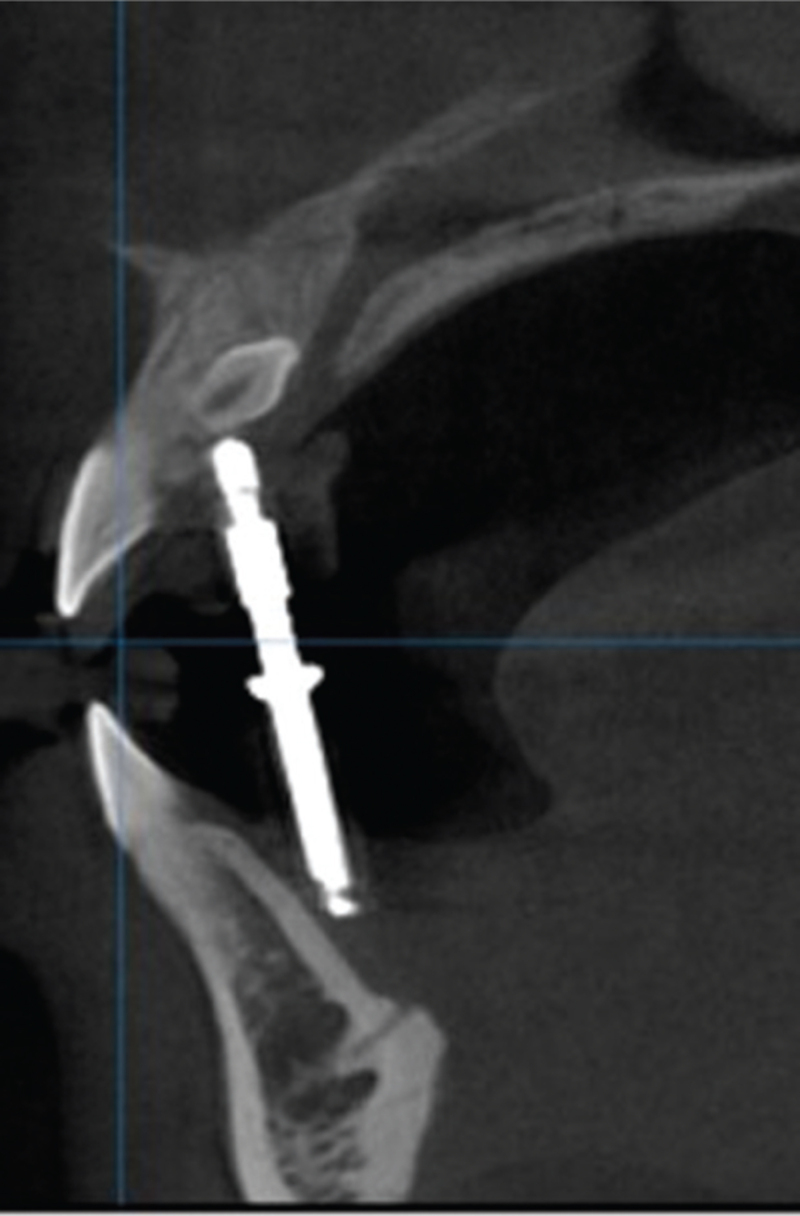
The guide ring was used to determine the depth of the fissure bur, in order to remove bone, as seen under cone beam computed tomography.
